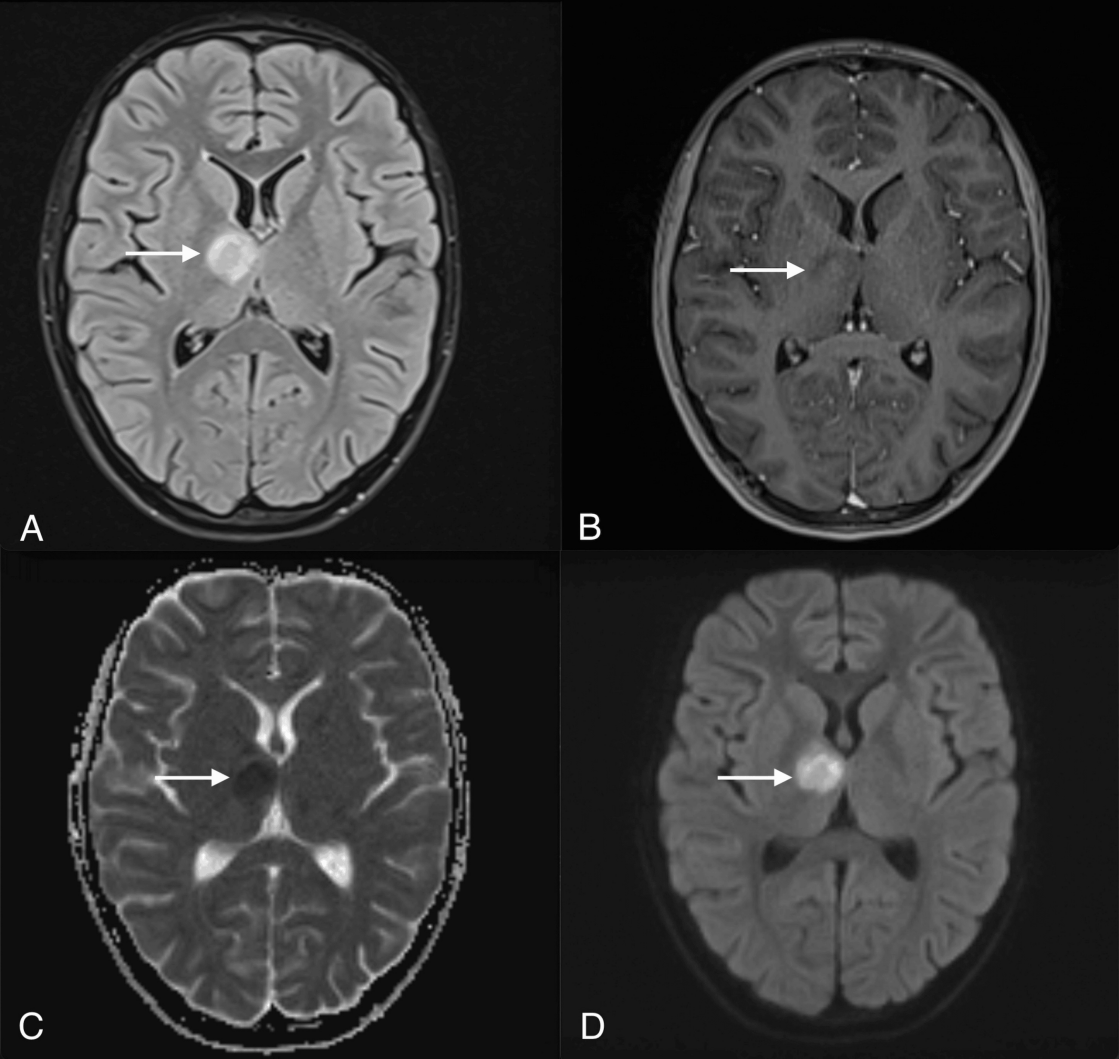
Day 5 from the onset of facial nerve palsy symptoms. Brain MRI at admission to the Pediatric Department demonstrates a pathological oval lesion in the right thalamus and internal capsule, hyperintense on T2 FLAIR (A) with trace enhancement on post-contrast T1-weighted imaging (B). Restricted diffusion is seen on the ADC map (C) and DWI (D). Arrows indicate the lesion. MRI, magnetic resonance imaging; ADC, apparent diffusion coefficient; DWI, diffusion-weighted imaging
